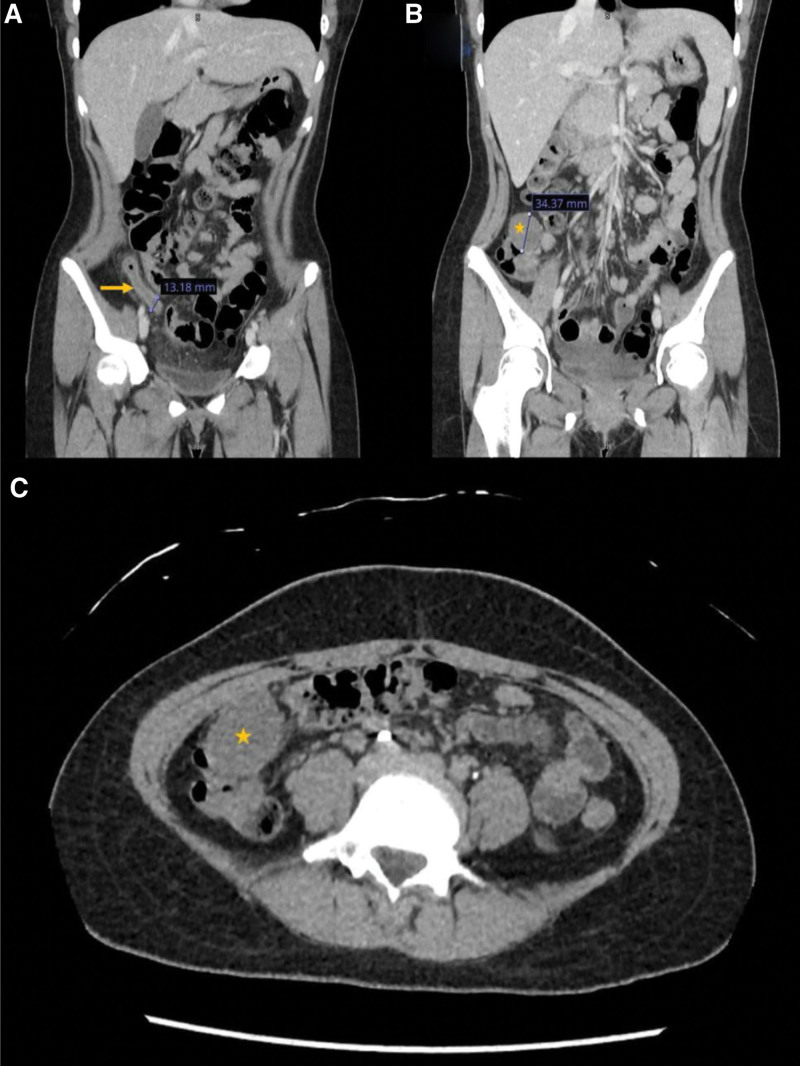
CT scan of the abdomen (A) Coronal view showing inflamed appendix (arrow) with enlarged thick appendix and peri-appendiceal fat stranding. (B, C) are coronal and cross sectional views respectively showing the cecal mass (star).
